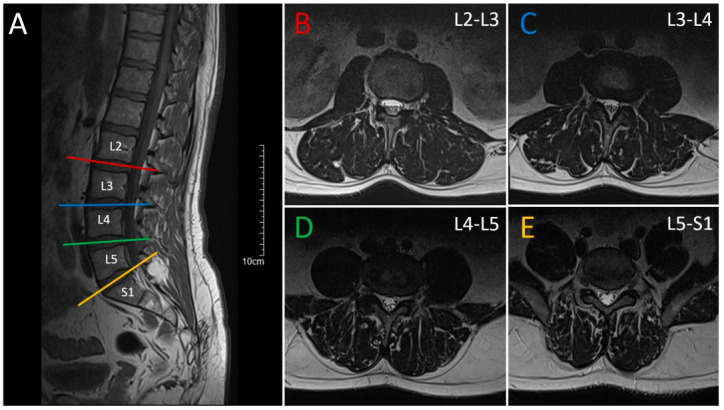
Magnetic Resonance Imaging (MRI) of the lumbar spine. (A): T1-weighted sagittal MRI showing spinal cord, degenerative changes in the lumbar spine with multilevel bulging of the intervertebral discs, osteochondrosis and lipoma at the level of L5-S1. Horizontal lines indicate the corresponding levels in the axial views. (B–E): T2-weighted axial MRI at different levels presenting the degenerative changes and intervertebral disc bulgings C6-C7—median disc herniation with compression of the dural sac and the spinal cord with myelopathy.
